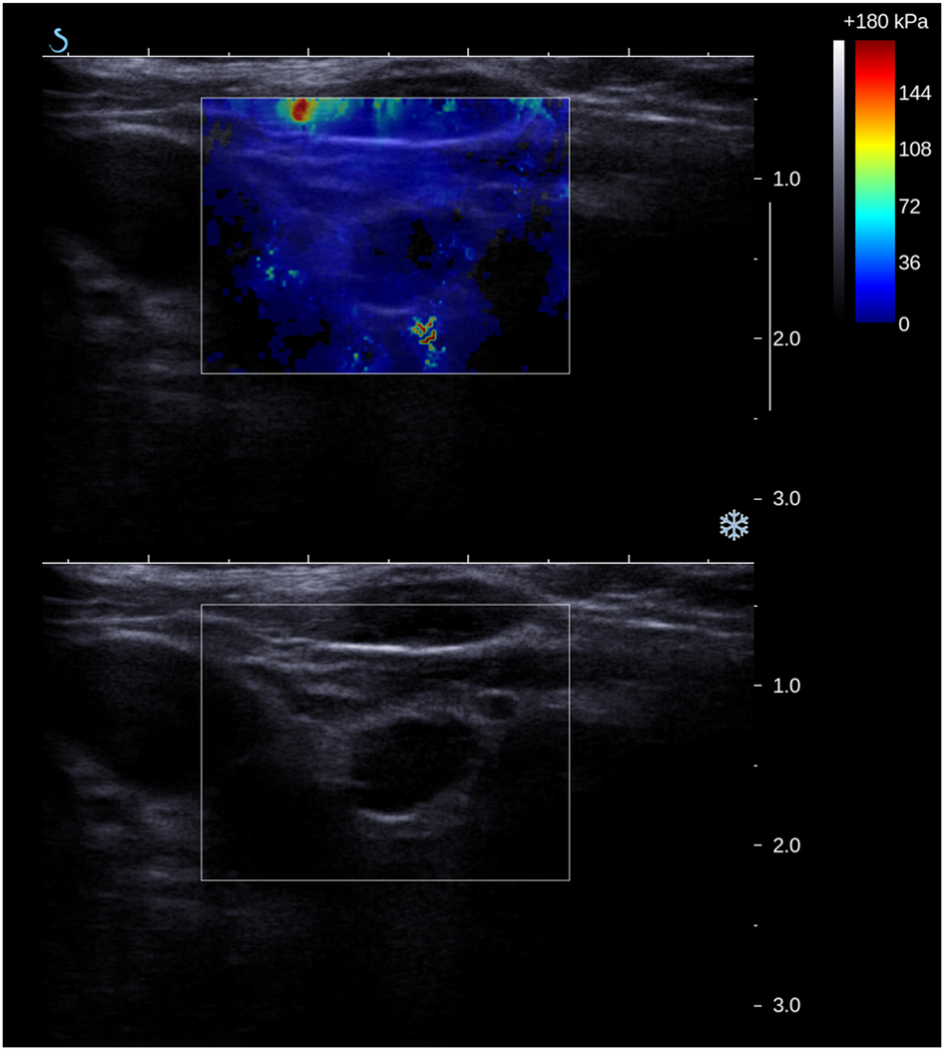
Example of parathyroid adenoma appearance in Shear wave elastography and conventional ultrasonography
